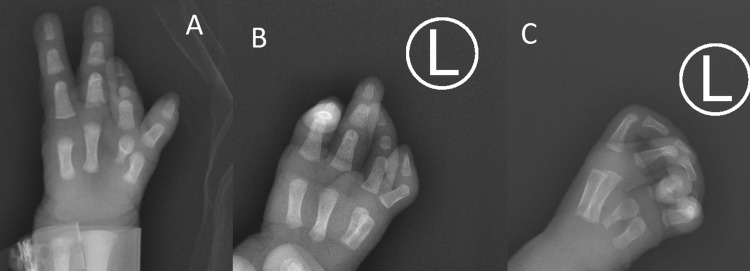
Initial X-ray of the left hand A: X-ray of the left hand taken shortly after birth. B and C: X-ray of the left hand taken at approximately one month of life.
